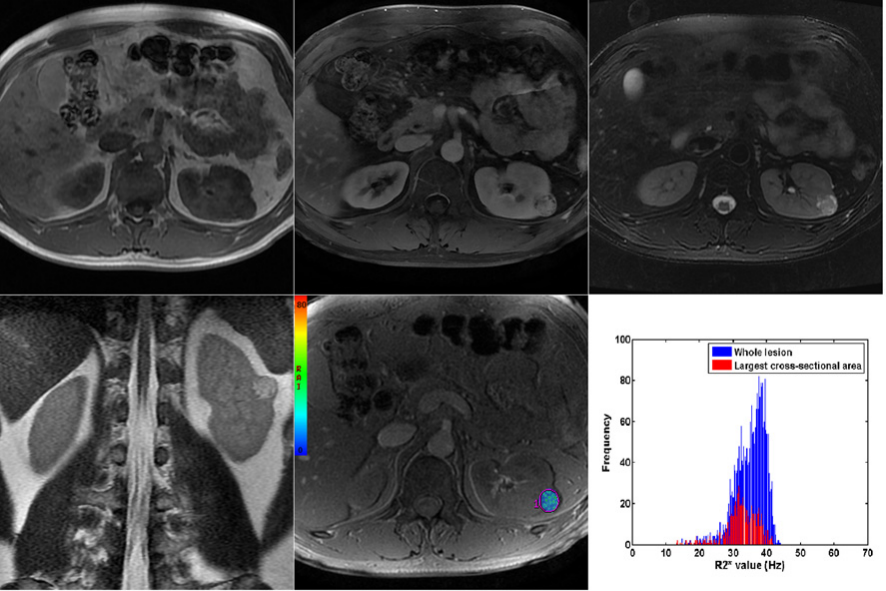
MRI scans of cRCC. (A) unenhanced T1-weighted in-phase (B) contrast-enhanced T1-weighted with fat suppression at nephrographic phase (C) T2-weighted image with fat suppression (D) coronal T2-weighted image (E) colour rate of spin dephasing (R2*) map, mean R2* value of renal cell carcinoma and histogram of R2* value derived based on largest cross-section and the whole tumour (F).
